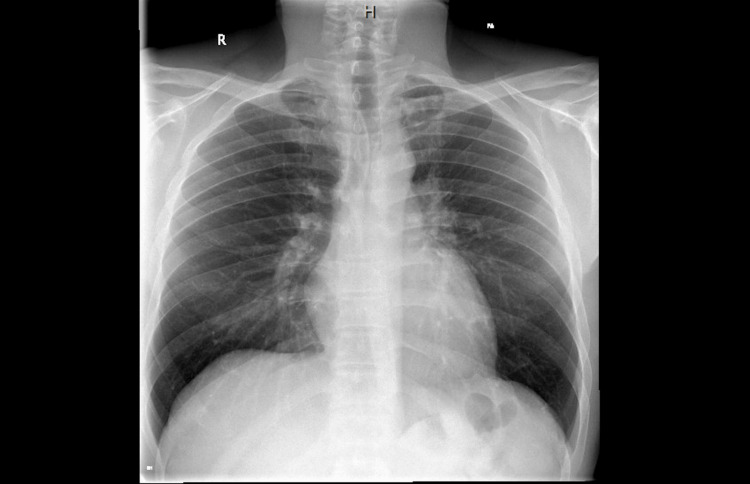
Initial X-ray of the chest Taken on April 14, 2022.
